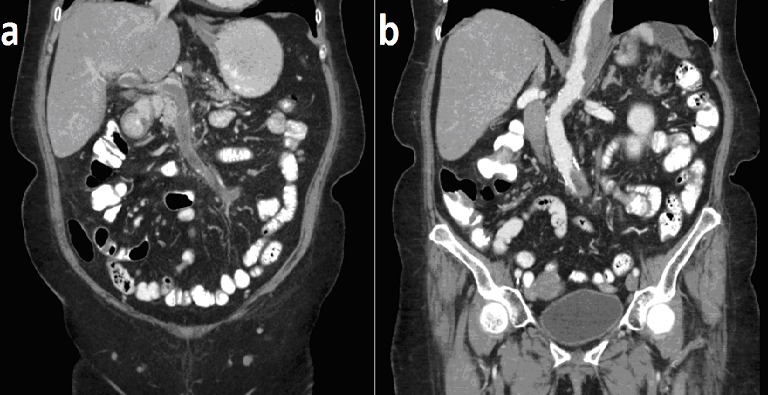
(A) shown are images of the CT scan of the patient with oral contrast medium, without and; (B) with intravenous contrast medium, showing a chronically dissected Stanford B thoracoabdominal aneurysm with a mural thrombus, thrombosis of the portal and superior mesenteric veins and a splenic infarct
